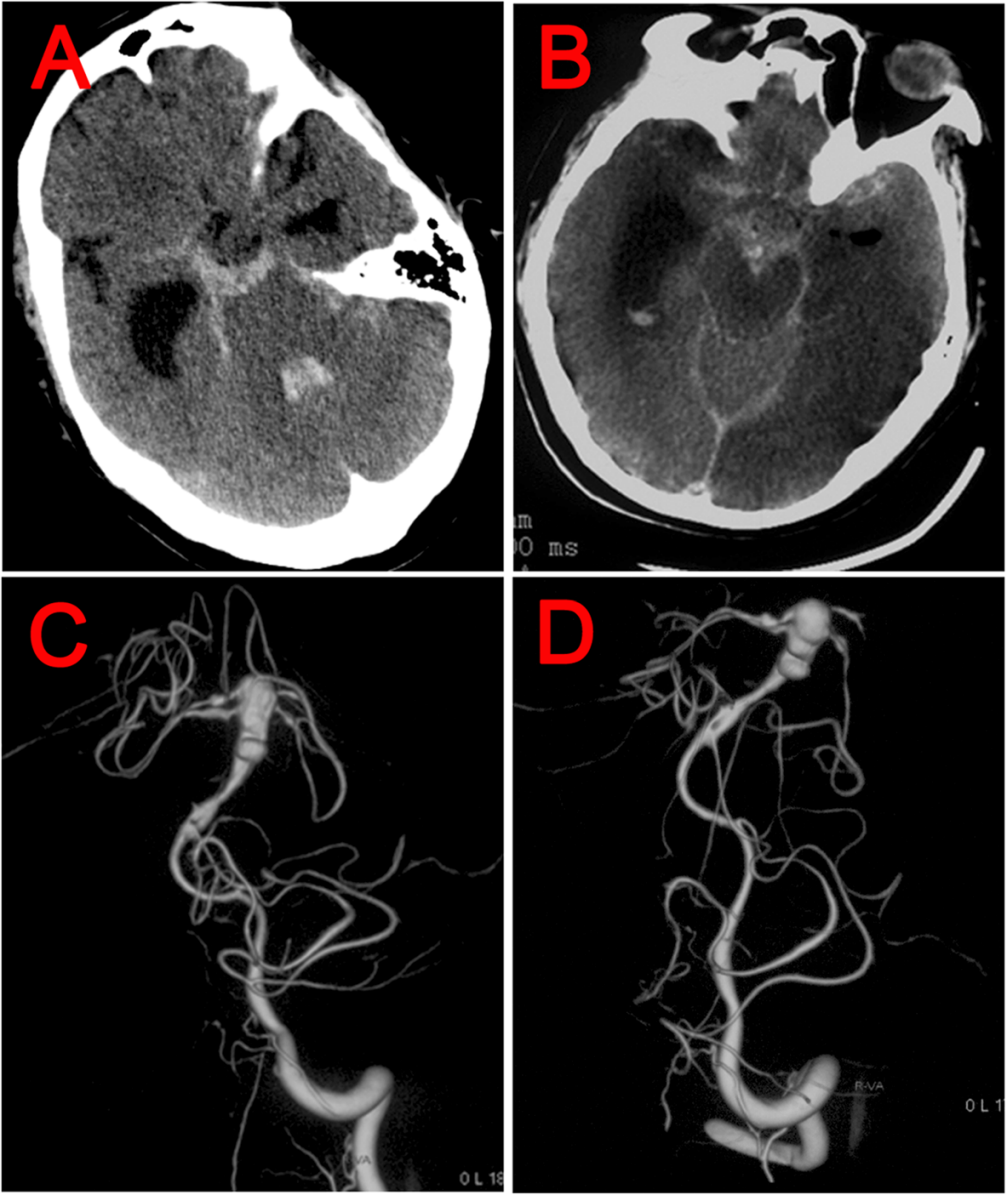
a Brain CT scan performed immediately after attack of sudden severe headache and loss of consciousness showed SAH at prepontine cistern and fourth ventricle. b Brain CT rechecked in the next day after SAH showed SAH was aggravated. c-d DSA showed an enormous fusiform aneurysm at the trunk of BA, which gave rise to some aneurismal secondary cysts. Moreover, original sites of bilateral SCA and PCA were also involved
